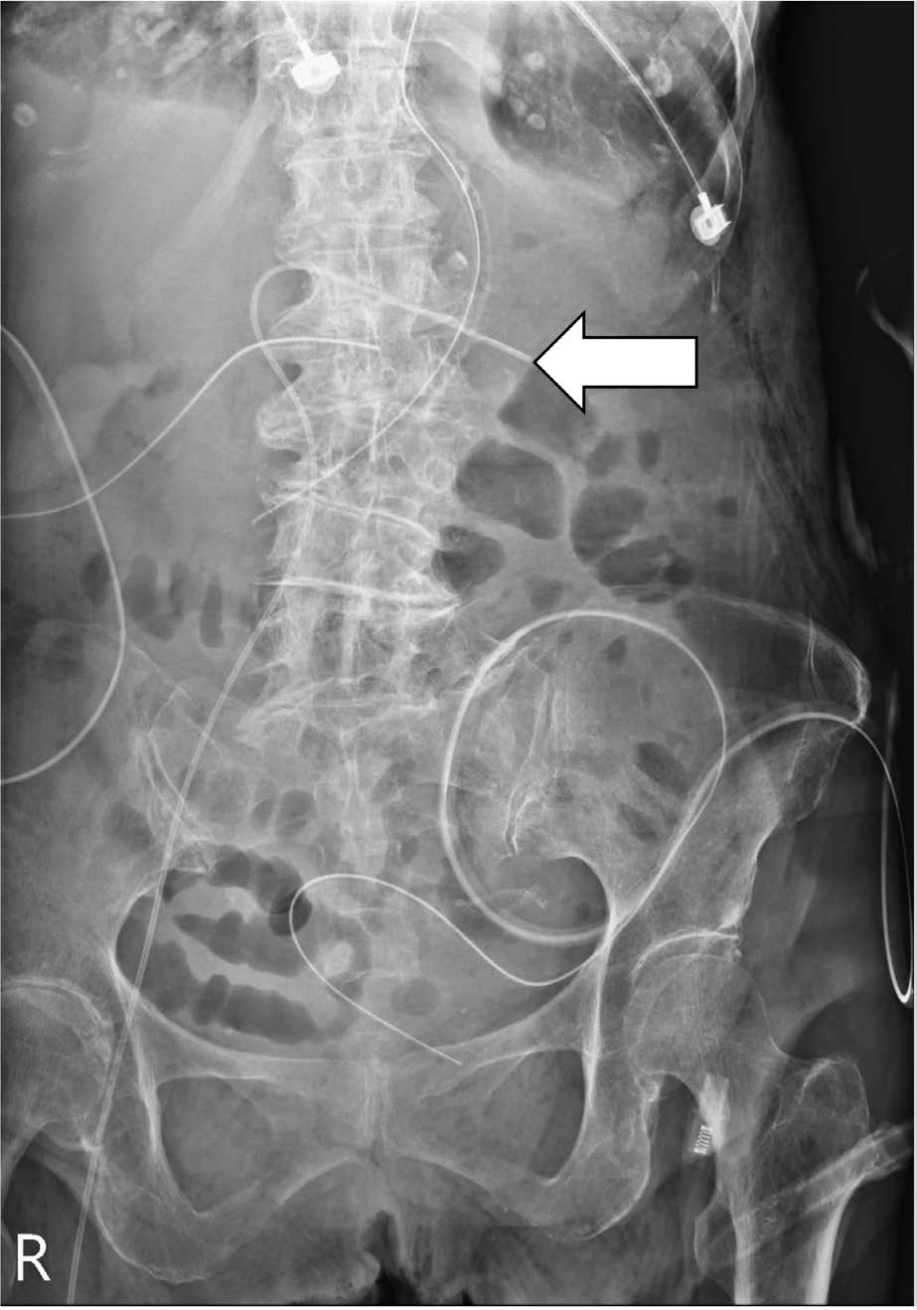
Postprocedural abdominal X-ray showing misplacement of the catheter tip in the contralateral renal vein (arrow).
